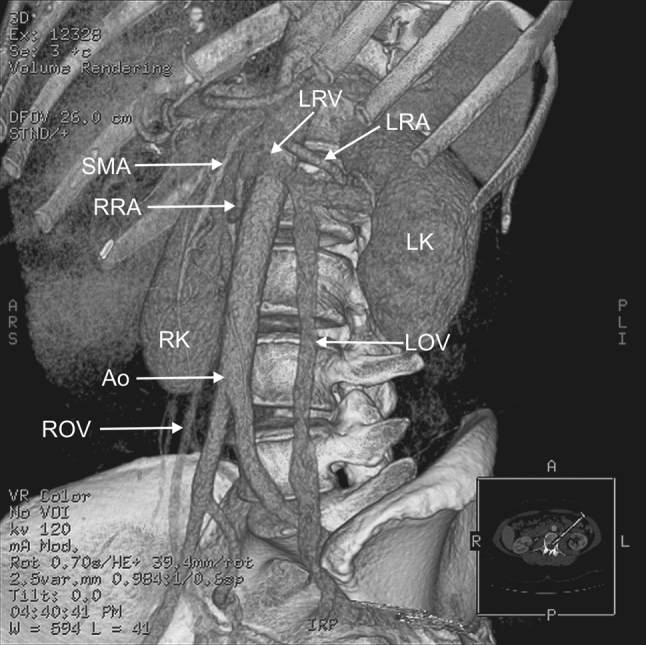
Three-dimensional CT reconstruction of the structures of the abdomen. *Ao* abdominal aorta, *LK* left kidney, *LOV* left ovarian vein, *LRA* left renal artery, *LRV* left renal vein, *SMA* superior mesenteric artery, *RK* right kidney, *RRA* right renal artery, *ROV* right ovarian vein
